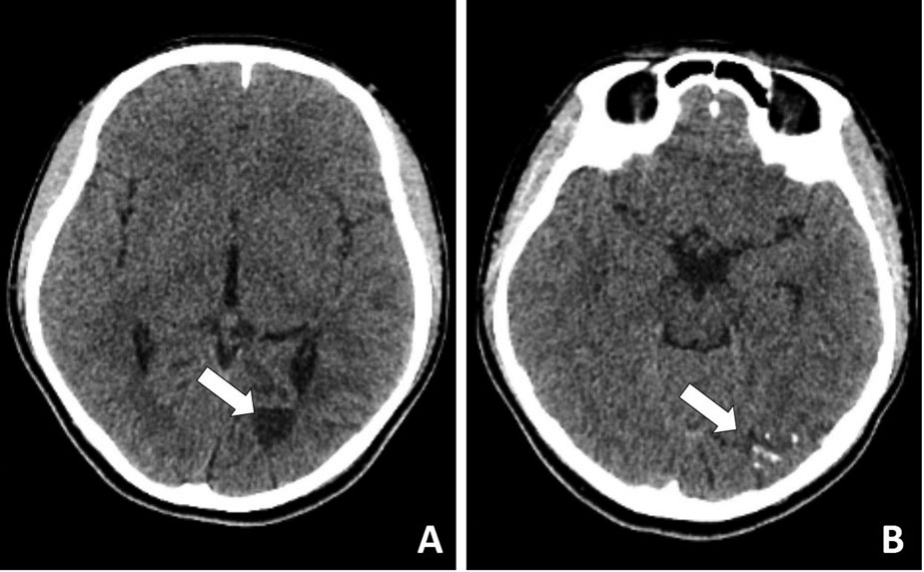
Computed tomography scan of the brain revealed an area of hypodensity in the region of the left lateral ventricle and left occipital lobe.
